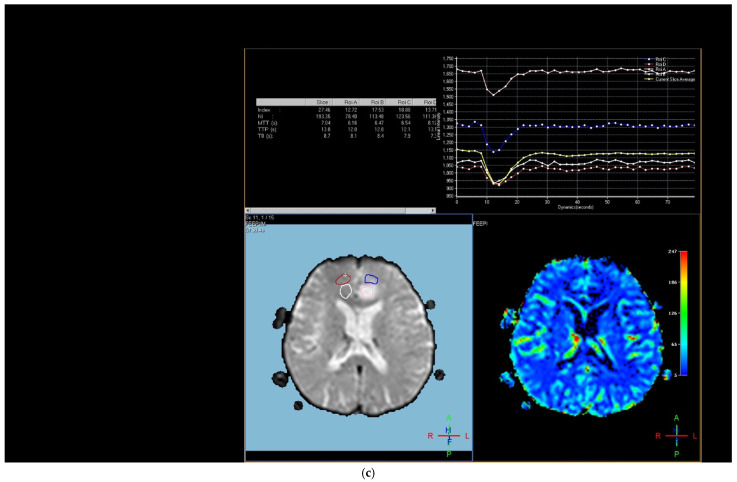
Brain magnetic resonance imaging (MRI) of a 52-year-old male with a low-grade glial tumor revealed (a) increased signal on a T2-weighted image, (b) hypoperfusion with a rCBV of 1.08 in perfusion-weighted imaging, Pink ROI is from tumor and white ROI is from contralateral parenchyma. and (c) a total of four ROIs—one from the peritumoral region (in blue), one from the contrast-enhancing tumoral area (in pink), and two from the contralateral tumor-free white matter areas corresponding to these regions (in white and red) in perfusion-weighted imaging.
